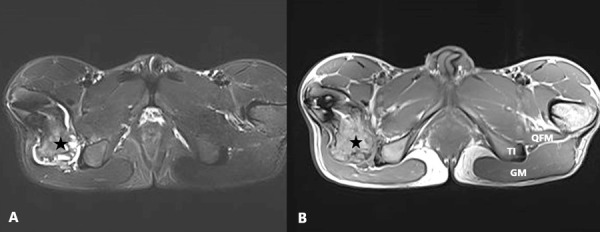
The hip MRI of the 32-year-old female patient. FSE T1W axial image (A) and fat saturation FSE T2W axial image (B). *Osteochondroma* (asterisk) causing severe *QFM* compression by narrowing the *ischiofemoral distance* on the right. Normal ischiofemoral distance and *QFM *(shown by black line and arrow) on the left. *GM: Gluteus maximus, TI: Tuber ischium, QFM: Quadratus femoris muscle, F: Femur.*
